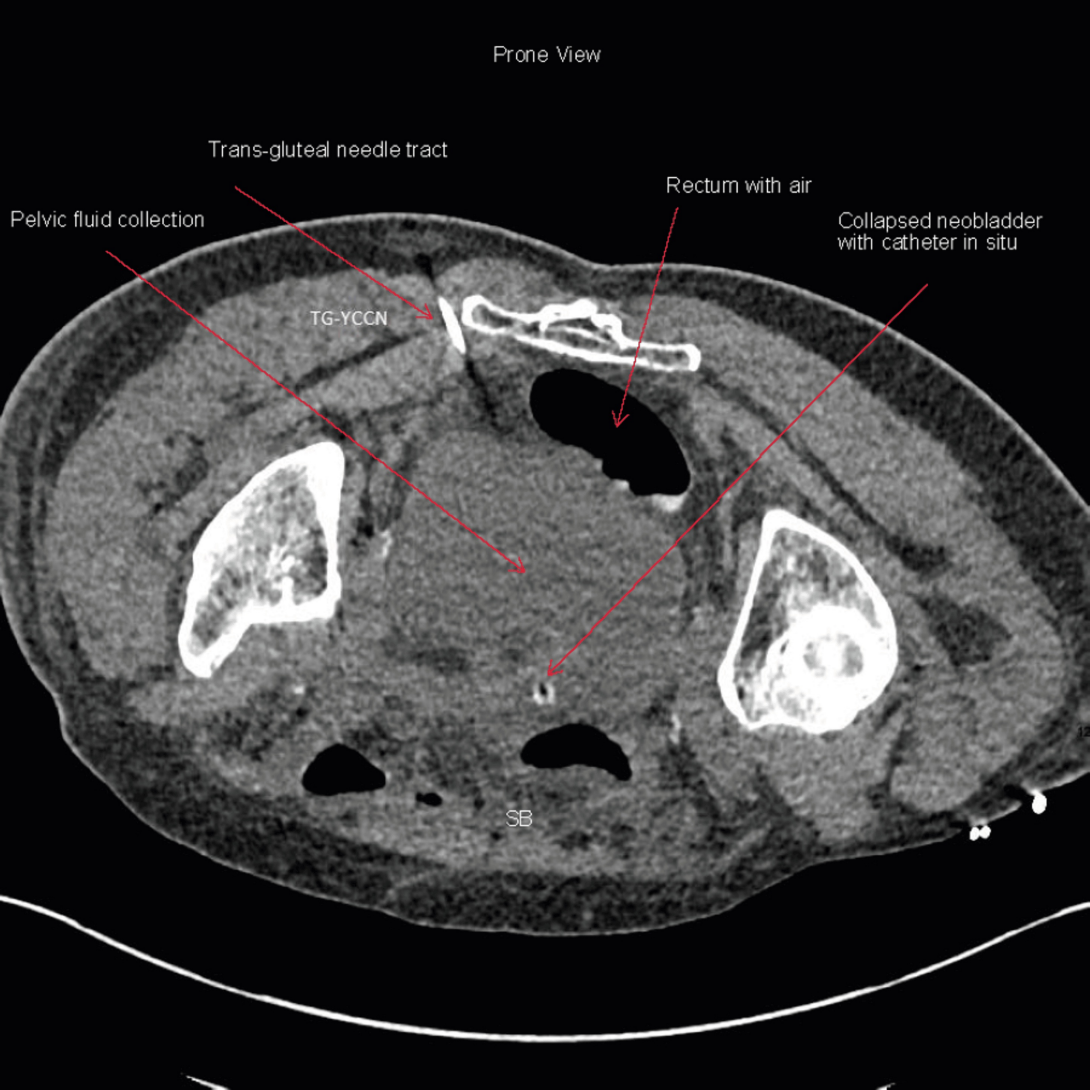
Axial prone CT showing trans-gluteal entry of CT-guided insertion of Yueh Centesis Catheter Needle (Cook Medical, Limerick, Ireland) to insert the pigtail drain into the pelvis TG-YCCN: Trans-gluteal Yueh Centesis Catheter Needle
